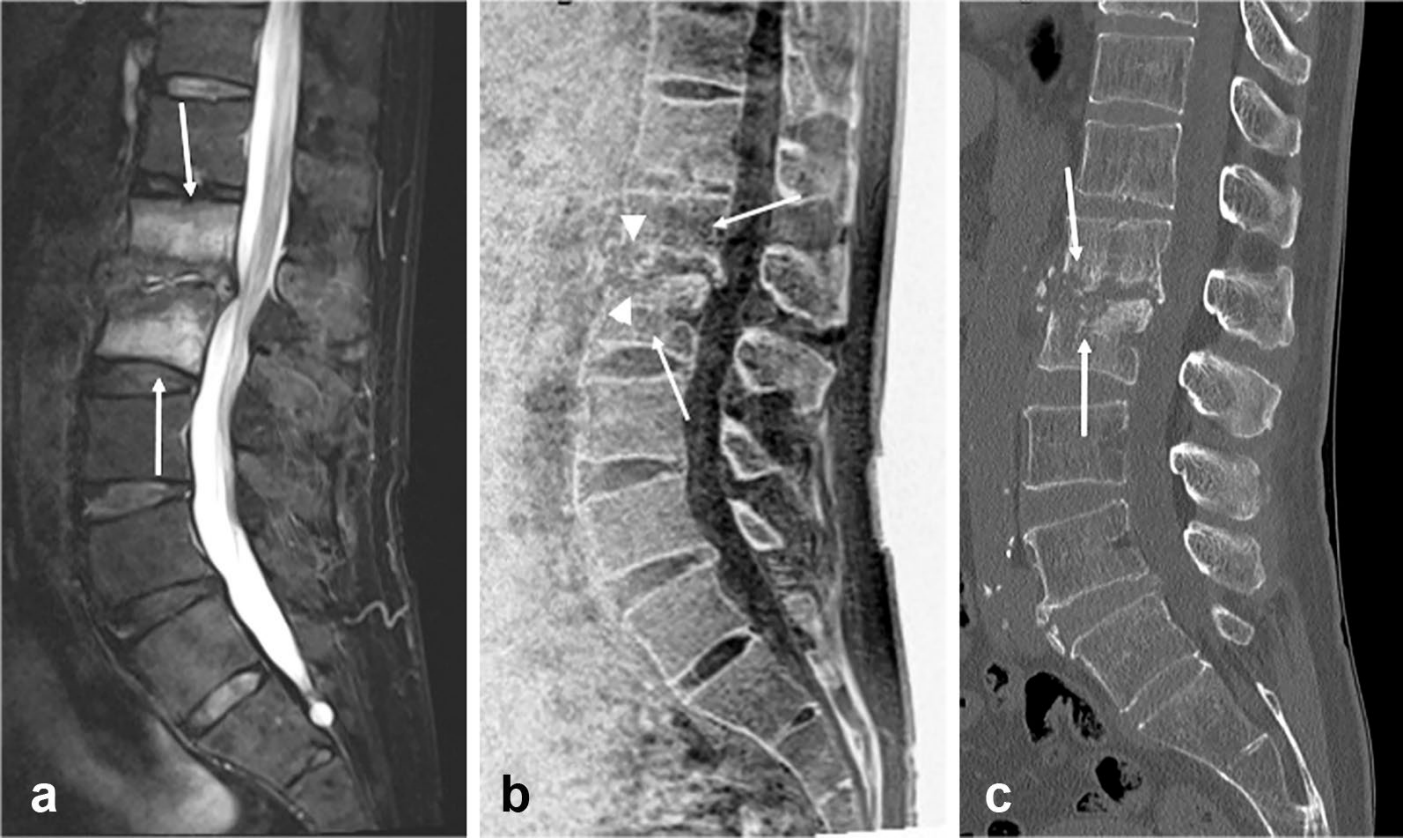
Pyogenic spondylodiscitis. Sagittal fat-suppressed T2-weighted MR image (a) of a 64-year-old male patient shows abnormal hyperintensity in L1 and L2 vertebrae and the flattened L1-2 disc due to spondylodiscitis (arrows). Sagittal MR bone image (b) clearly shows the disrupted endplates of L1 and L2 vertebrae (arrowheads) and sparse trabeculae (arrows). Sagittal CT image (c) shows the corresponding destruction of the endplates of the L1 and L2 bodies facing the narrowed L1-2 disc (arrows)
